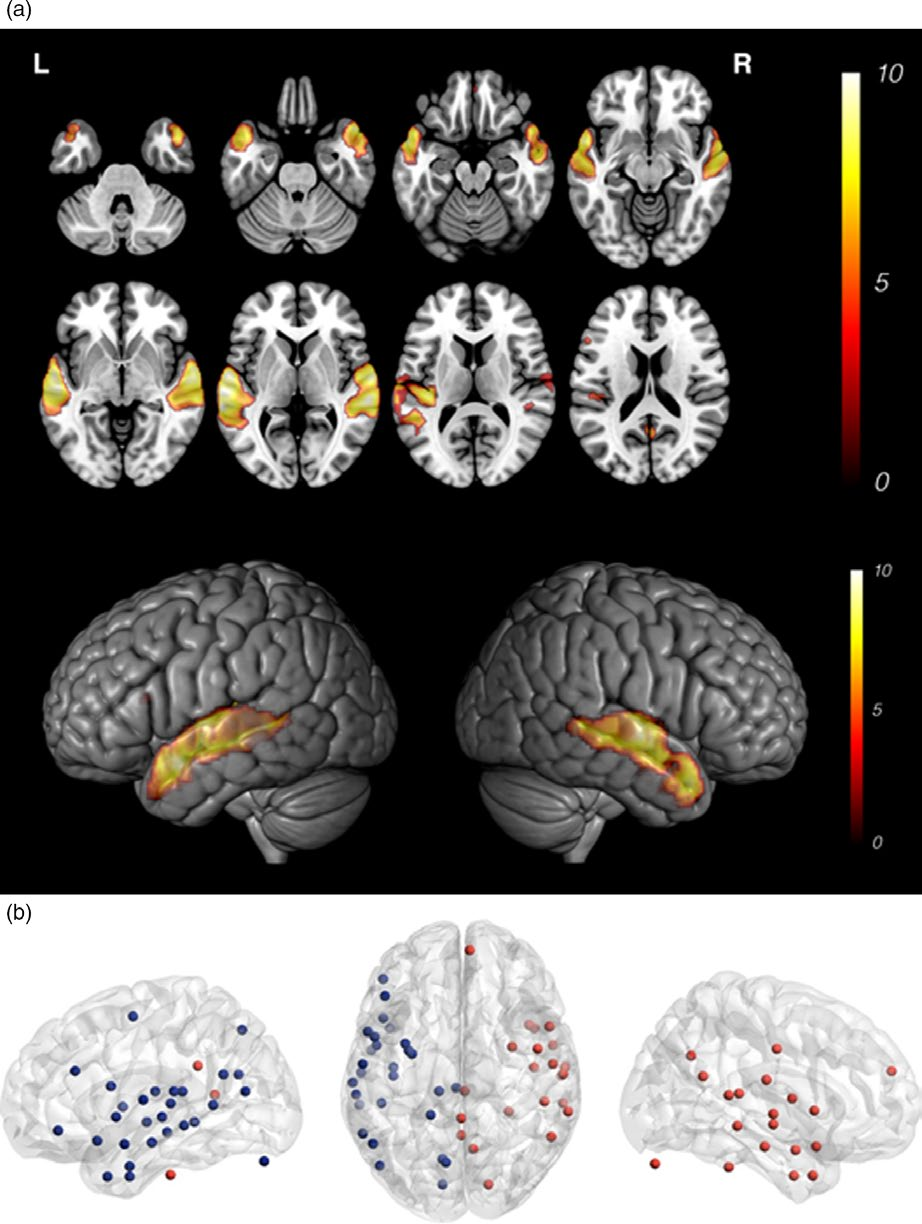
Functional MRI activation maps and network nodes. (A) Eight axial slices showing typical bilateral temporoparietal activation for stories versus noise (*p* < .001, *n* = 30, 15 TC and 15 EPT) and 3D surface rendering showing activation from lateral perspective. (B) The activation map is parcellated to produce the “nodes” for network analysis
